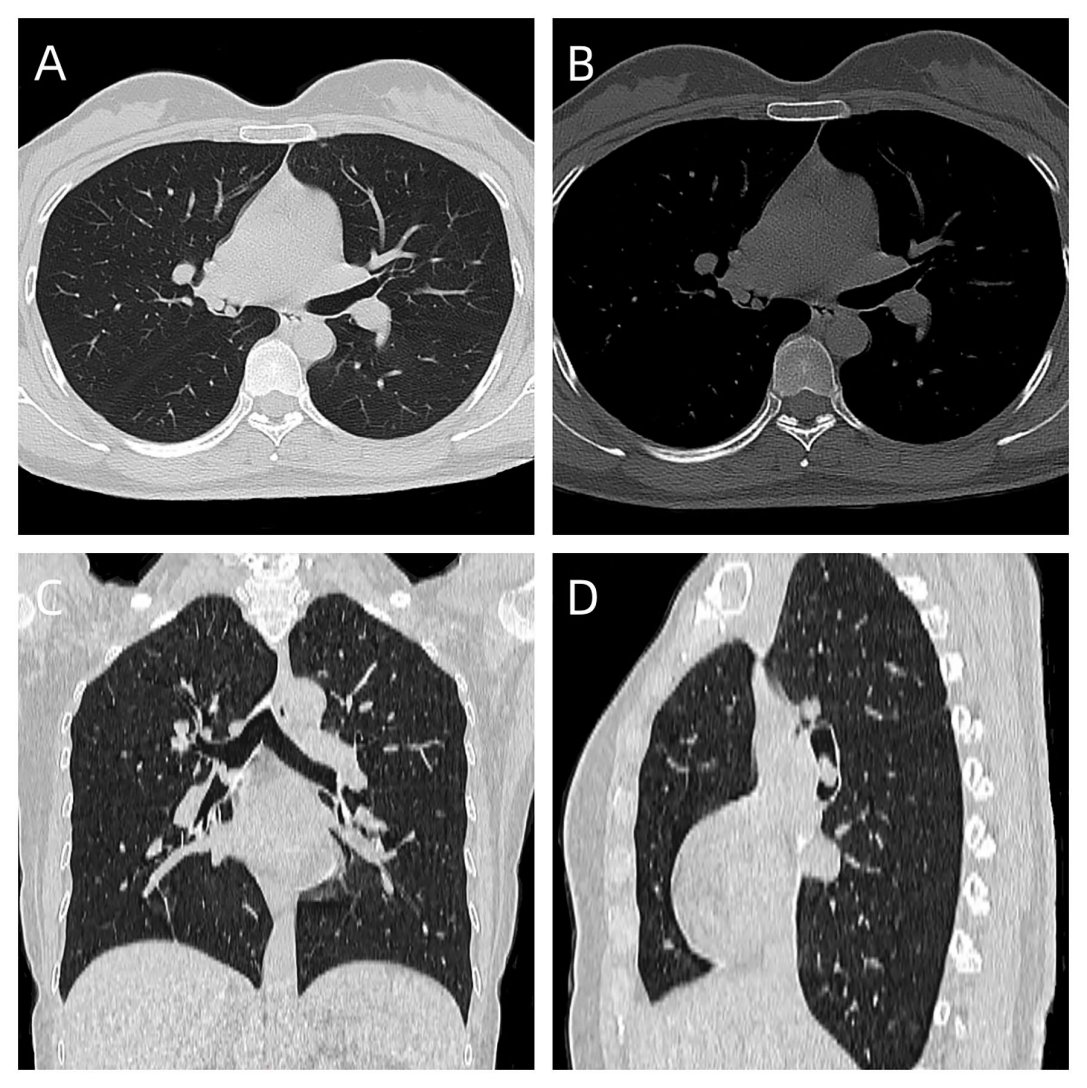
(A) axial lung window, CT showed multiple polypoid lesions in the middle bronchus of the right lung; (B) axial bone window; (C) coronal scan; (D) saggital scan.
